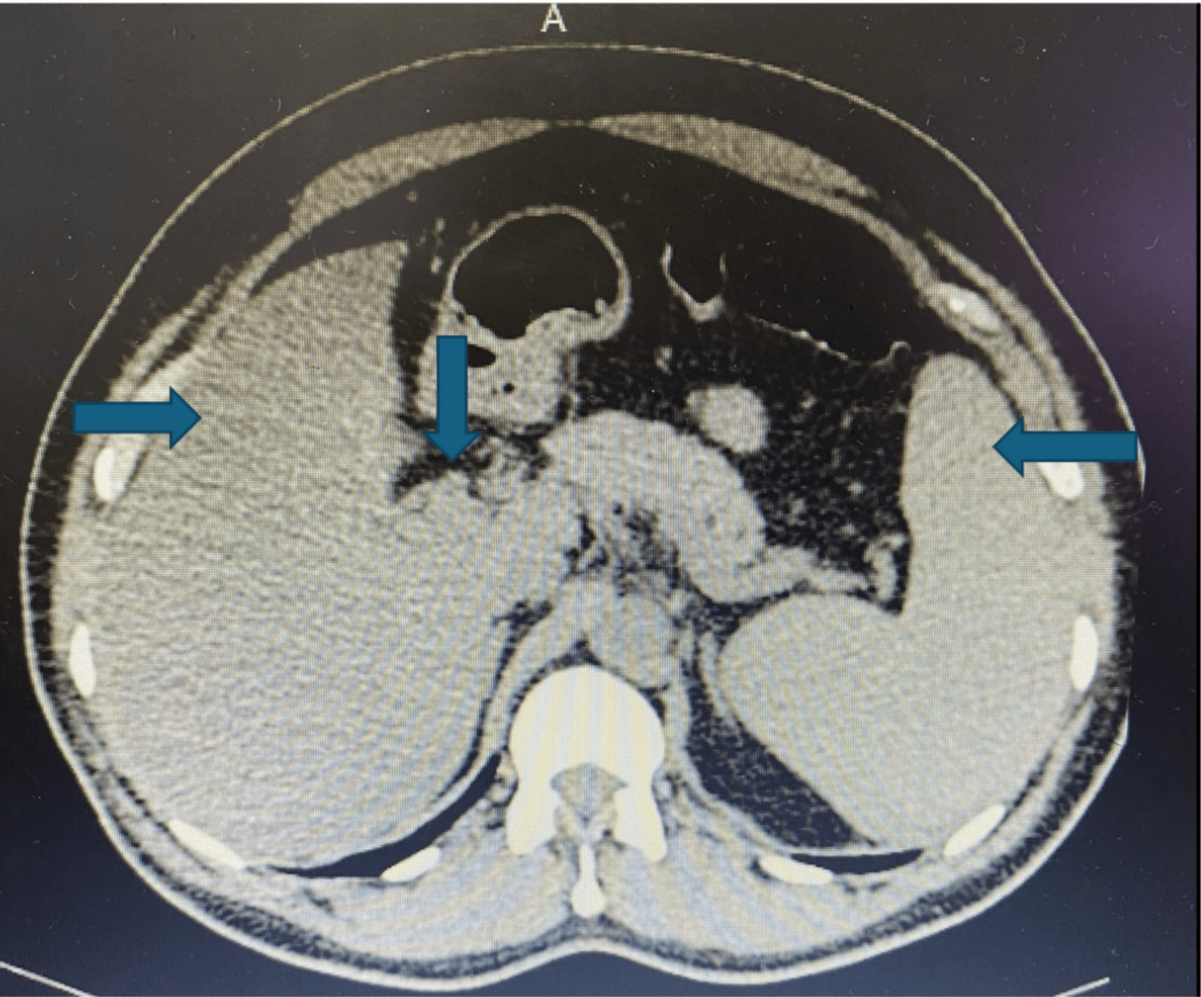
Computed tomography scan of the whole abdomen showing diffuse fatty liver, mild splenomegaly with dilated portal vein.
